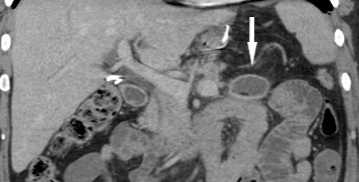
Coronal CT post-i.v. contrast 1 week post laparascopic Roux-en-Y gastric bypass using bioabsorbable prosthesis with ﬁbrin glue ﬁxation to prevent a Petersen’s space hernia. Funnel shaped structure (arrow) in Peterson’s defect corresponds to the Gore BioA tissue reinforcement mesh
